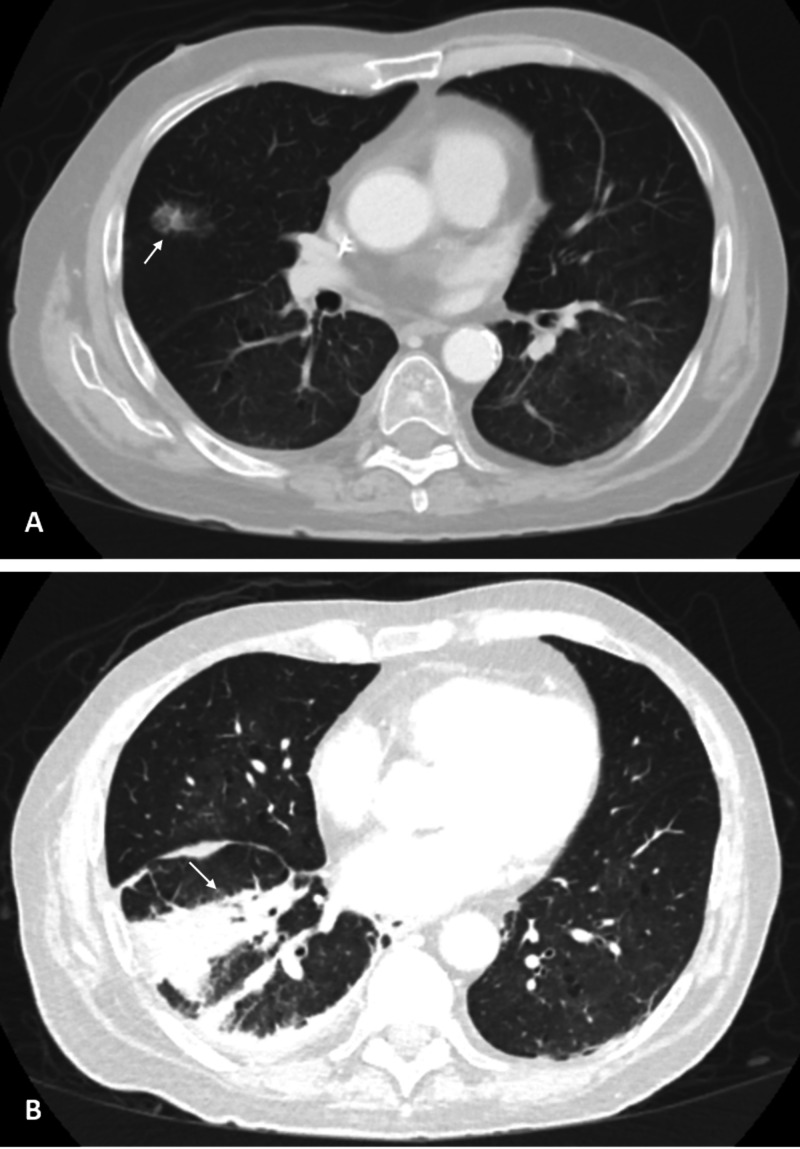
Chest imaging studies A: CT of the chest showing nodule in the right middle lobe with ground-glass and spiculated solid components. B: right lower lobe opacity with ground-glass and airspace components with a peripheral component and linear component extending centrally associated with pleural effusion/thickening.
